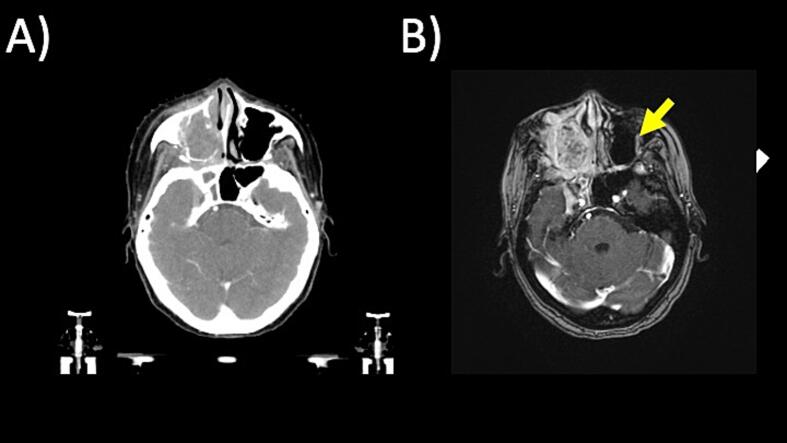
A patient with a right maxillary sinus adenoid cystic carcinoma noted on MRI sim to have unsuspected contralateral perineural tumor spread of the left infraorbital nerve. (A) CT and (B) MRI sim images are shown.
